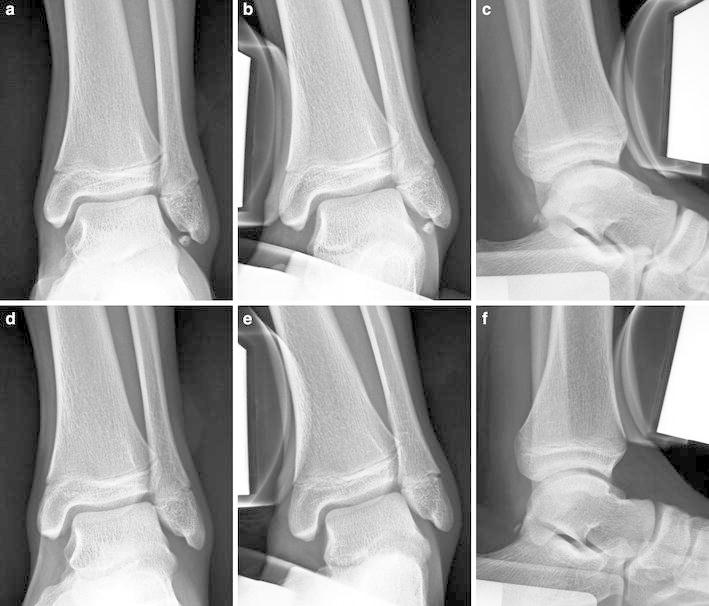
Preoperative radiographs (a–c) and postoperative radiographs (d–f) (Case 19). Anteroposterior radiograph (a) showing a small ossicle (5 mm in diameter). Varus stress radiograph (b) and anterior stress radiograph (c) showing no instability of the ankle. Anteroposterior radiograph (d) showing a ossicle excised. Varus stress radiograph (e) and anterior stress radiograph (f) showing no increase of ankle instability
